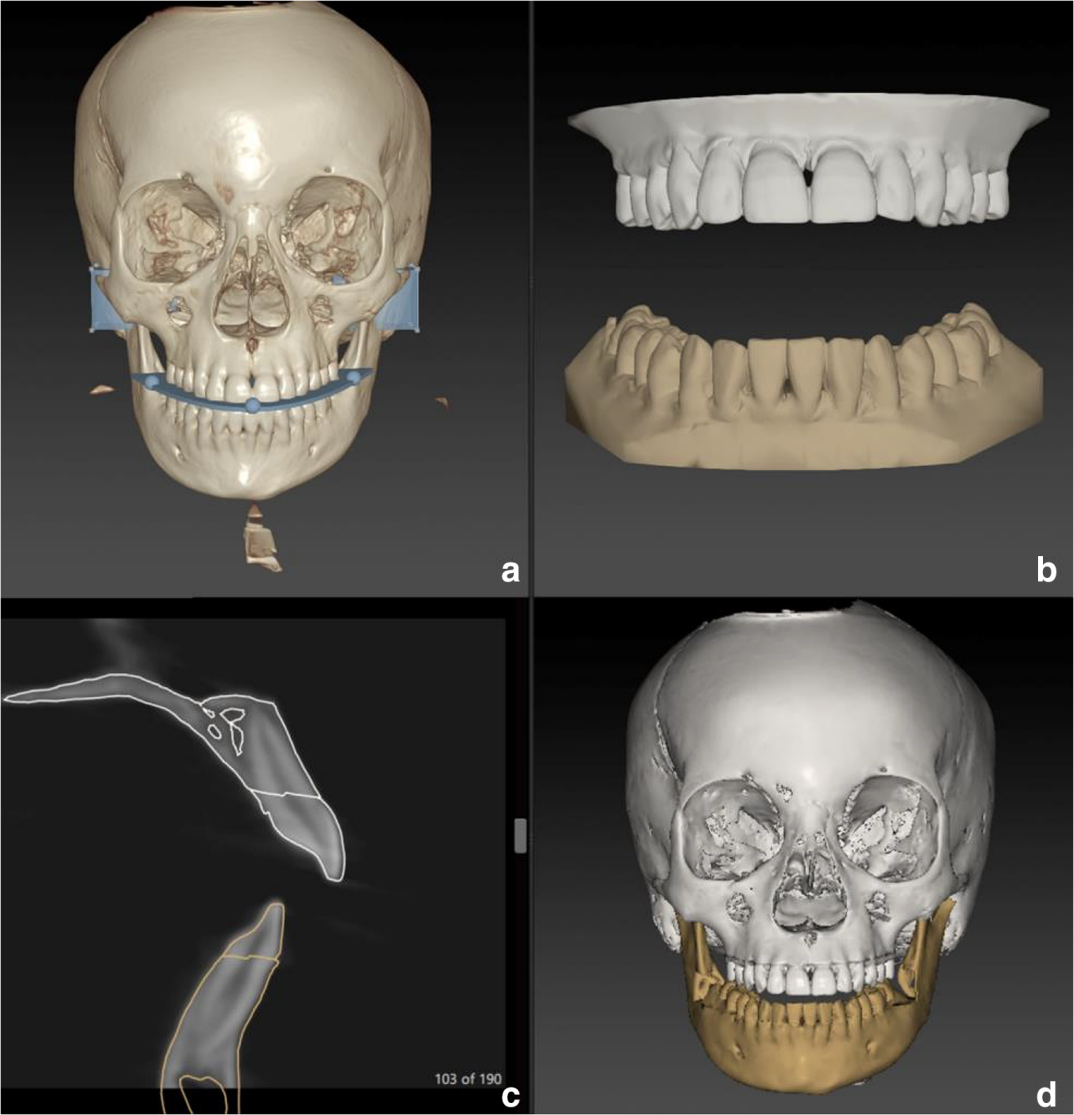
Overview of the fusion process in the IPS CaseDesigner®. a Three-dimensional CBCT skull model in the IPS CaseDesigner® software with the left and the right condyle indicated as well as the occlusion plane. b Intra-oral scans of maxilla and mandible. c Cross-sectional view of the 3D CBCT skull model and fused intra-oral scans. d Frontal view of the result of the fusion of the intra-oral scans in the CBCT
